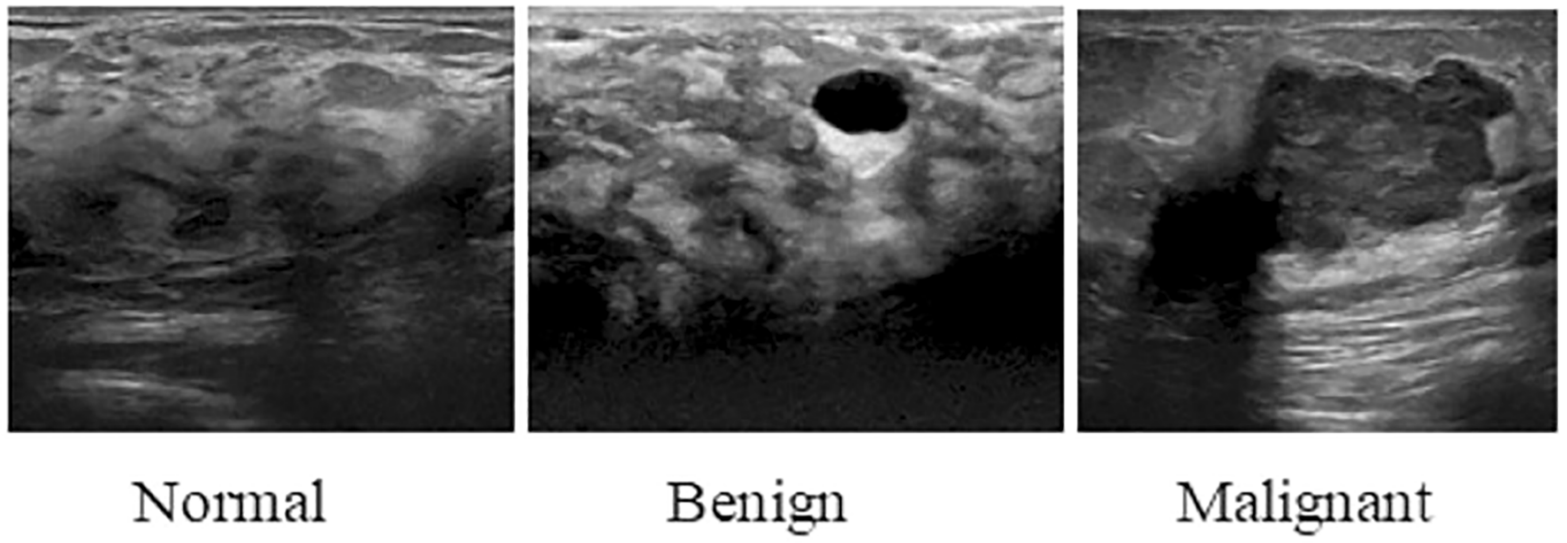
Ultrasound scans of breast in different conditions (210).
